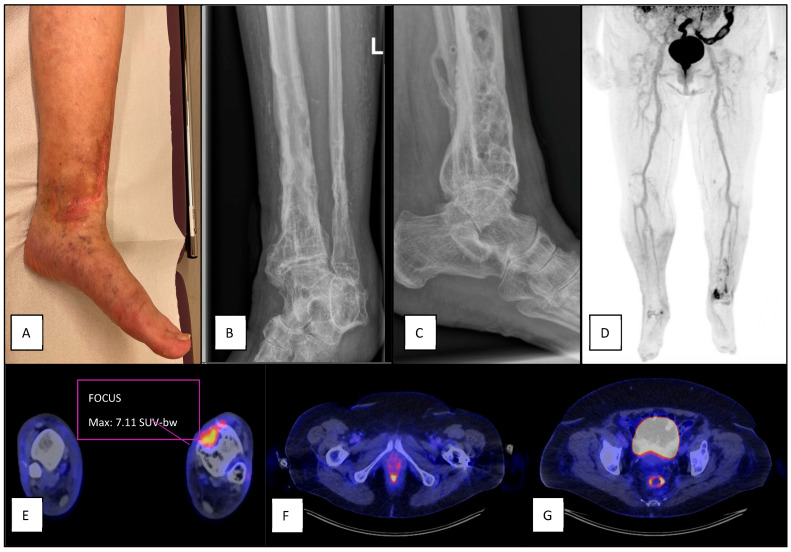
Patient presented 18 years after sustaining a distal tibia fracture for which a plate and screw osteosynthesis was performed. The plate and scews were later removed. The patient presented with local pain and erythema (A), but no clinical confirmatory signs. Plain radiograph showed extensive post-traumatic bone deformity (B,C). FDG-PET/CT showed increased FDG-uptake at the distal tibia (D,E). No increased FDG-uptake was seen in inguinal (F) or iliac (G) lymph nodes. Intra-operative cultures were positive for *Corynebacterium propinquum*.
